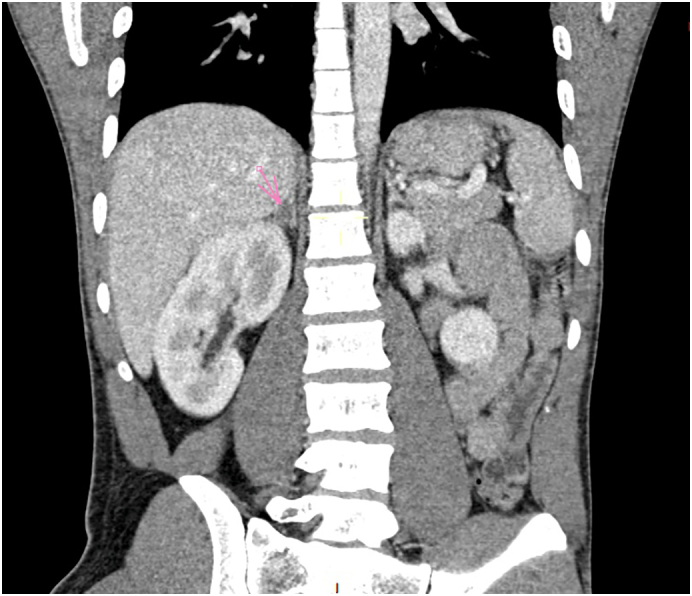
Coronal view of abdominal CT scan was performed and showed a right adrenal cortical adenoma.
